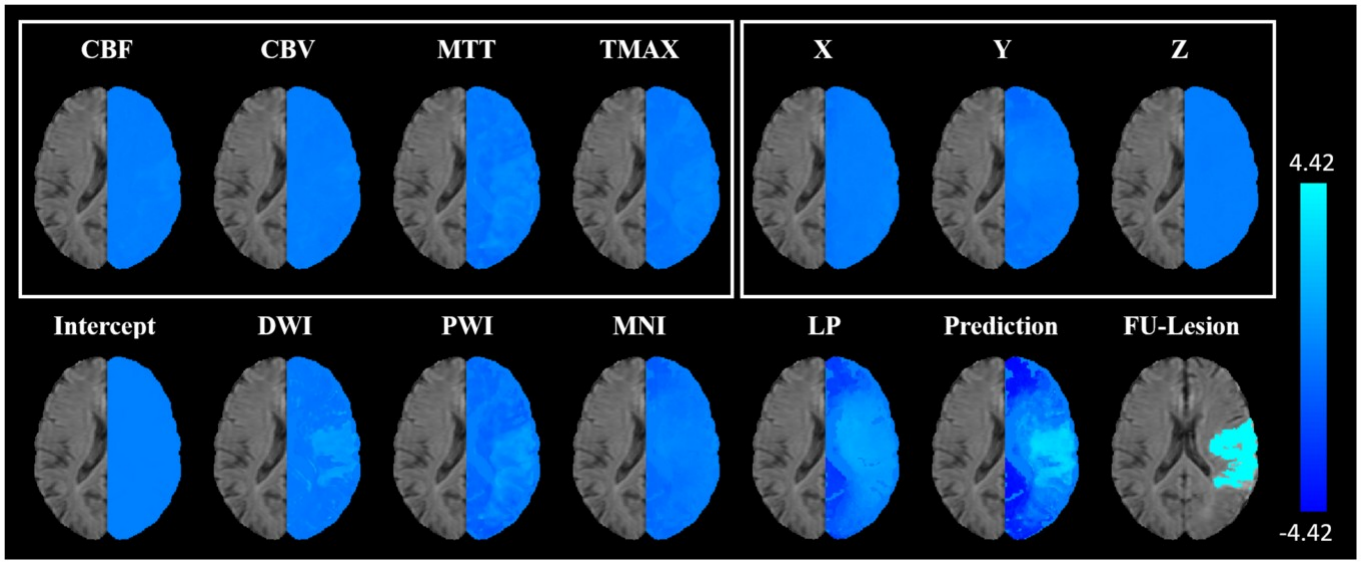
Shapley value decomposition. Shapley value decomposition of the predictions from the XGB model incorporating DWI, PWI, MNI, and LP (setting 6). The sum of the intercept and the Shapley values for DWI, PWI, MNI, and LP correspond to the prediction of the follow-up lesion on the logit scale (bottom row). The Shapley values for PWI and MNI are themselves sums of the Shapley values of CBF, CBV, MTT, Tmax (PWI) and X, Y, Z (MNI; top row). Further addition of the MNI and LP Shapley value maps would lead to a high-level spatial Shapley value map incorporating all spatial features from the model. Similar Shapley value maps for the other three best XGB models per setting can be found in the online supplement.
